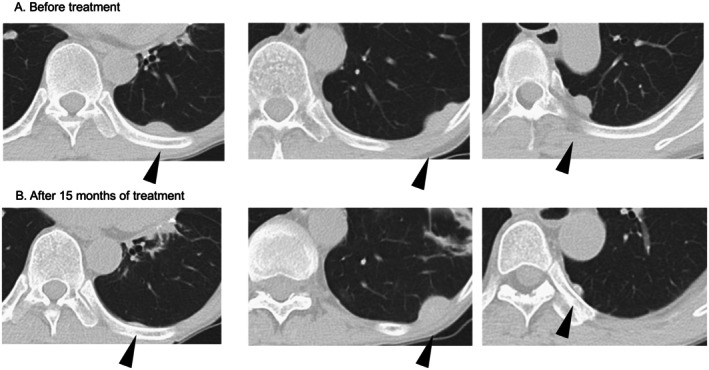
Abdominal computed tomography scan showing metastatic diseases before IO therapy and 12 months after. CT scan at diagnosis shows three pleural metastatic nodules (A). Fifteen months after the IO therapy, all metastatic lesions showed significant size reduction (B).
